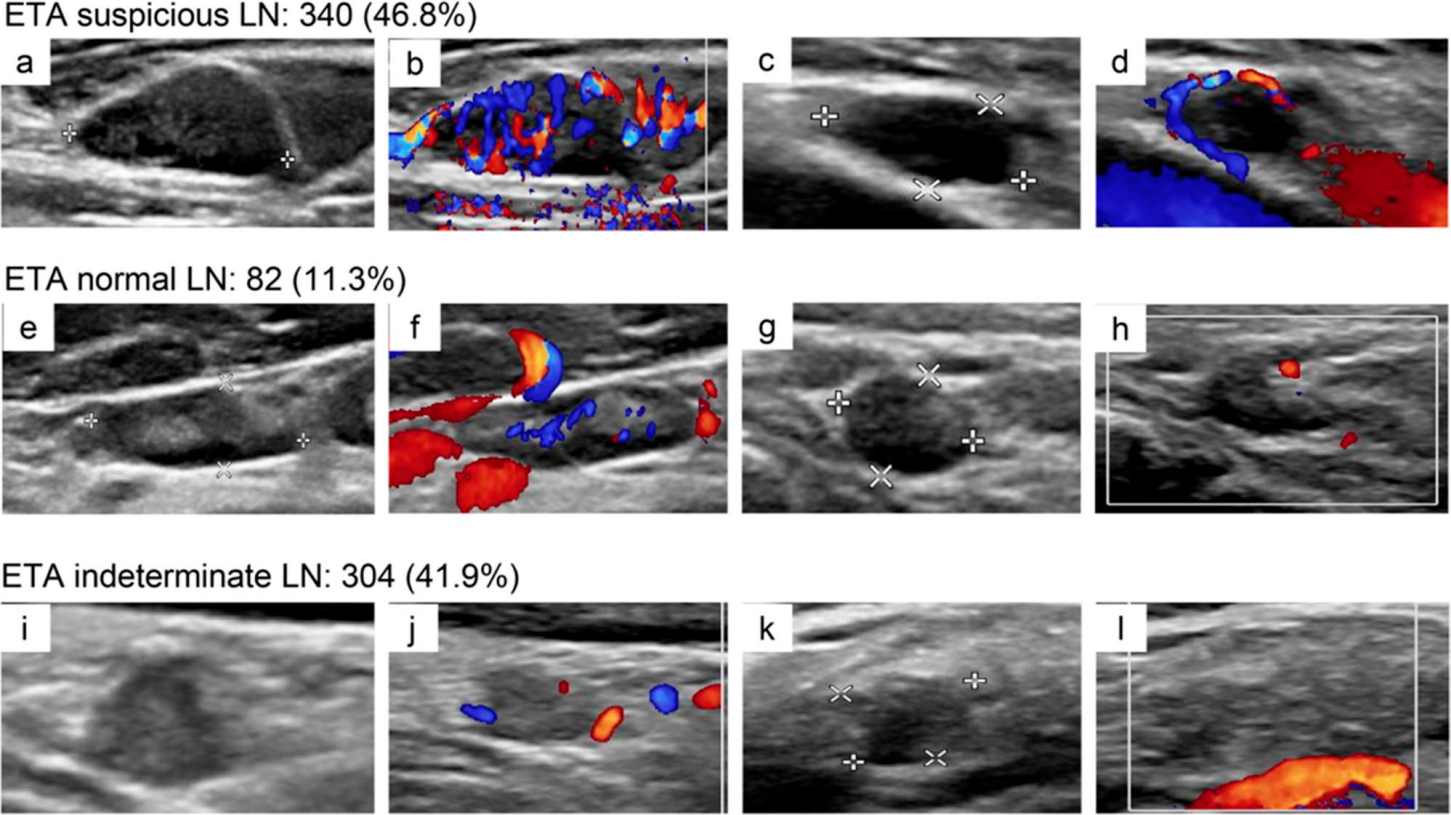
Examples of LNs in different ETA classifications. (a-d) ETA suspicious malignant LNs: (a, b) Hilum disappears with focal hyperechoic, and CDFI shows mixed and rich blood flow; (c, d) Hilum disappears and CDFI shows peripheral blood flow; (e-h) ETA normal LNs: (e, f) Hilum is clearly detected and CDFI shows hilar blood flow; (g, h) LN cortical is thickening with normal hilum and CDFI shows punctate blood flow. (i-l) ETA indeterminate LNs: (i, j) Hilum is unclear and CDFI shows punctate blood flow; (k, l) Hilum is unclear and no blood flow signal in CDFI. LNs: lymph nodes; CDFI: color doppler flow imaging; ETA: European Thyroid Association
